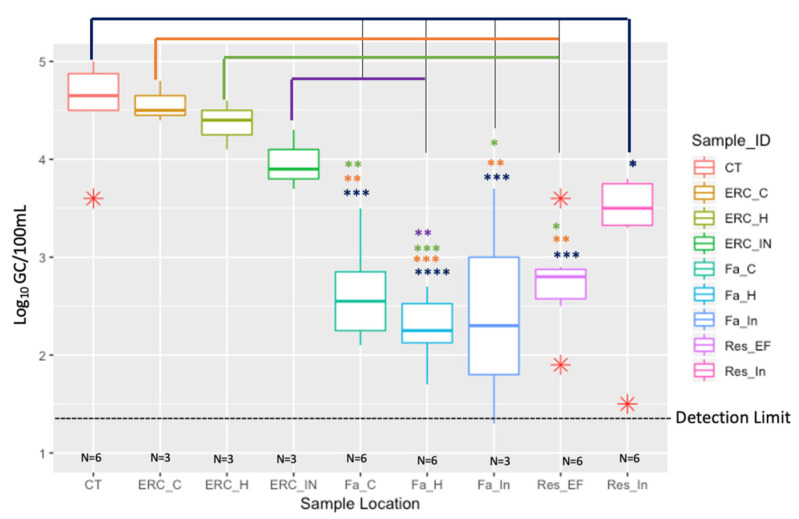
Comparison of *Legionella* spp. (23S rRNA) in the reservoir (RES_IN and RES_EF), buildings: Fa and ERC, and the Cooling Towers (CT). The water age (h) in Res_In (4.5 h), Res_EF (3.4 h), Fa (9.2 h), ERC (20.8 h), and CT (23.2 h). Description names are as follows: Res_In: Reservoir Influent; Res_Ef: Reservior Effluent; Fa_In: Fa Influent; Fa_H: Fa Hot-water tap; Fa_C: Fa Cold-water tap; ERC_In: ERC Influent; ERC_H: ERC Hot-water tap; ERC_C: ERC Cold-water tap; CT: Cooling Towers. The median of each measure is indicated by the thick colorful bar in each respective box; the first and third quartiles are represented by the bottom and top of the box, respectively; the red asterisks (*) show depicts the outliers within three sample types. The different asterisk (*, , *, ) colors represent the significant difference between sampling location and is as follows: blue: CT; orange: ERC_C; green: ERC_H; purple: ERC_IN. Detection limit was 1.3 Log_10_ GC/100 mL.
